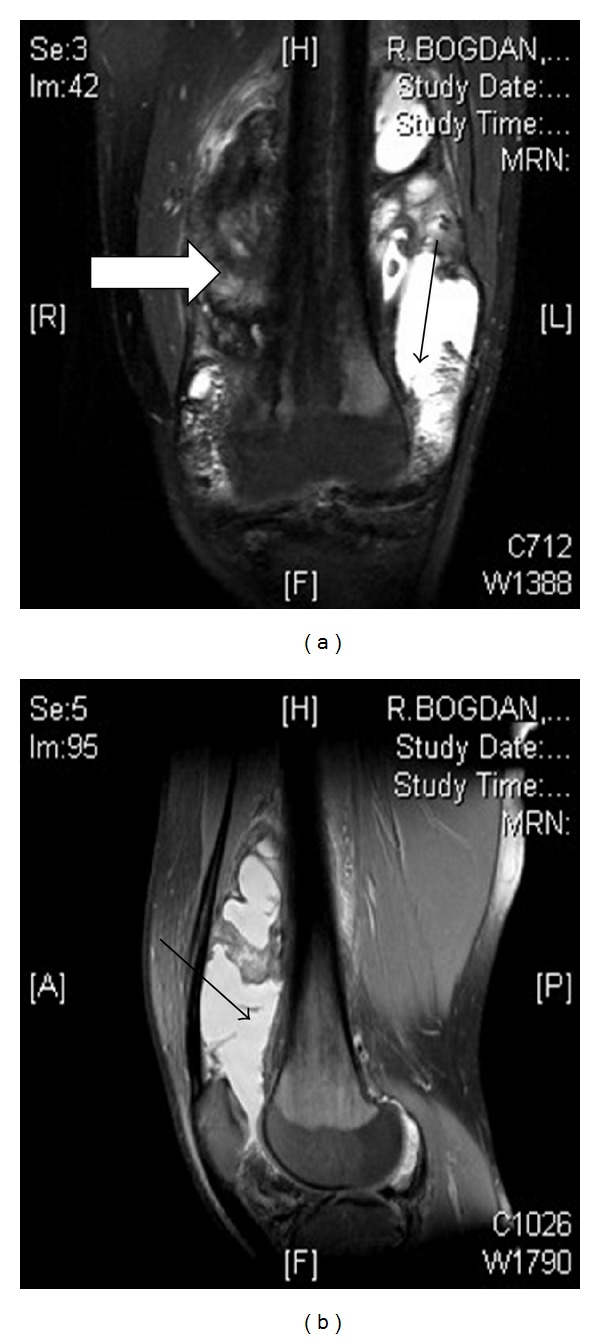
MRI of the knee joint of a 27-year-old haemophiliac. In the AP view (a) intra-articular blood can be noted in the lateral side of the joint (black arrow), while in the medial side a severe degree of synovitis can be seen (white arrow). In the lateral view of the MRI (b), the aforementioned hemarthrosis can also be noted (black arrow).
